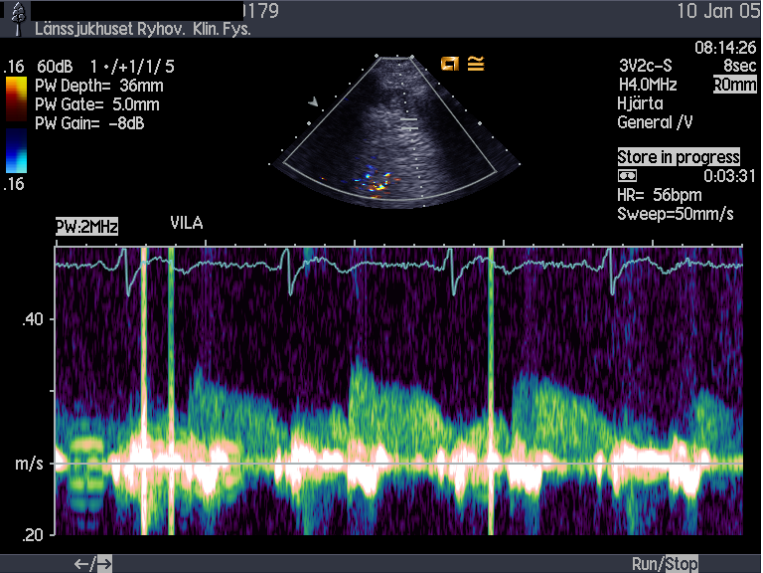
Spectral Doppler recording of LAD flow at rest.
